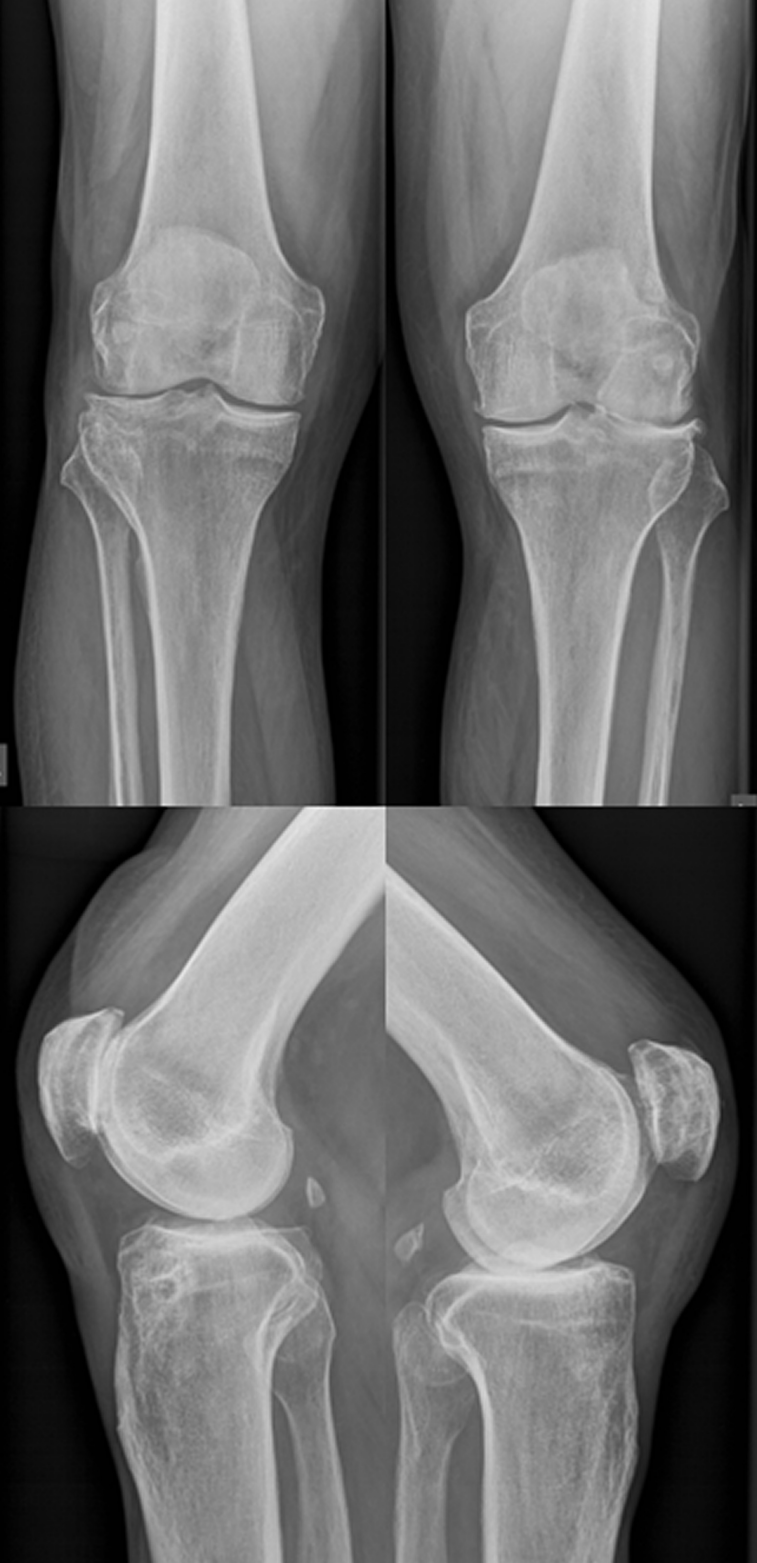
Preoperative radiographs of the knees. Tibiofemoral accentuated gonarthritis at the left side.
